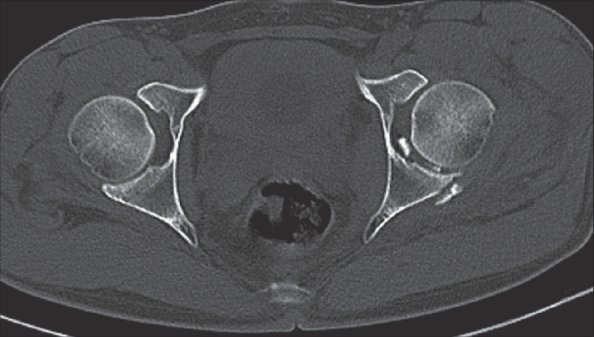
CT scan showing the retained fragments and fracture of the posterior lip of the acetabulum
